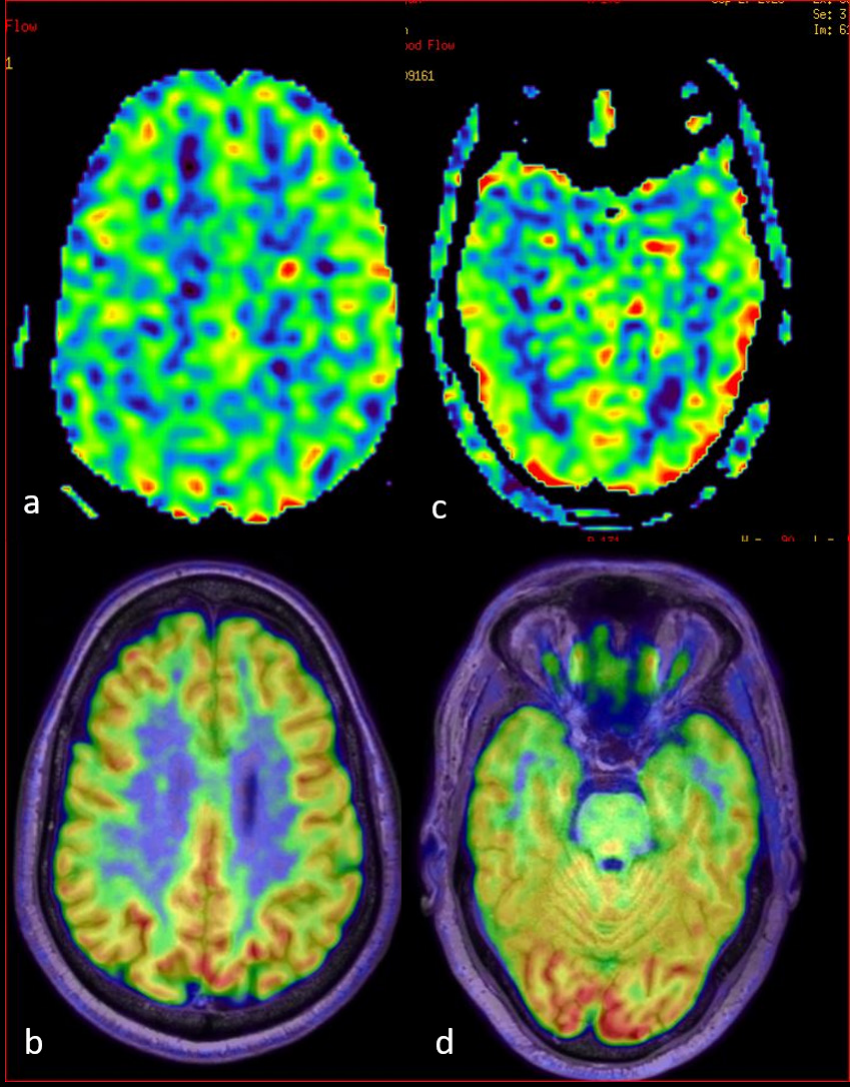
A 61-year-old with an ACE-3 score of 92 shows gross visual concordance between ASL (a, c) and PET (b, d) in areas of significant FDG-PET hypo-metabolism. ASL (a) and the corresponding PET-MR fusion image (b) show hypo-perfusion in the bilateral posterior frontal region (approx. Z score -4.2 on FDG-PET). ASL (c) and the corresponding PET (d) show hypo-perfusion in the bilateral anterior temporal region (approx. Z Score -2.2 on FDG-PET)
